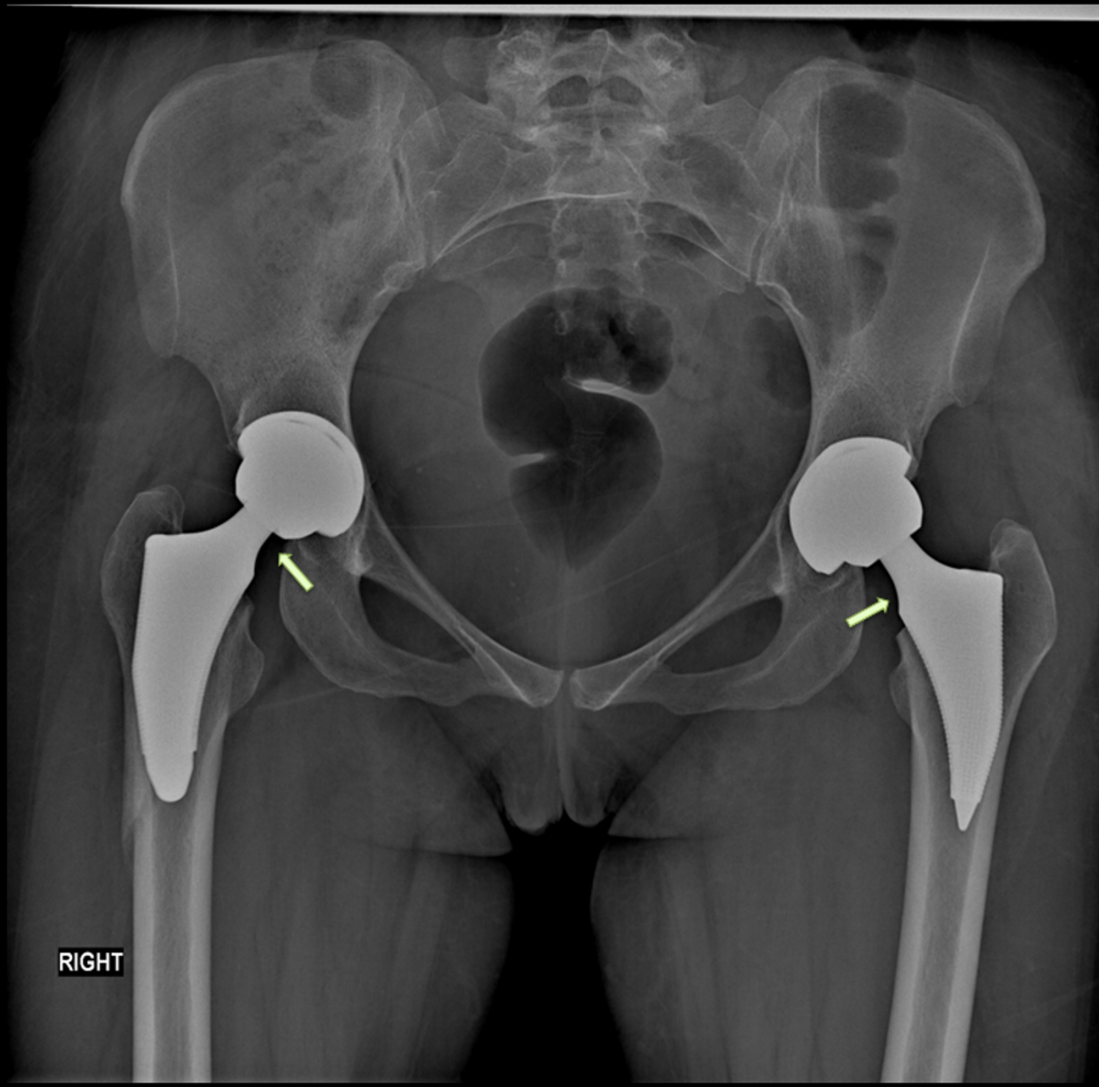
Postoperative radiograph of the pelvis showing bilateral total hip ceramic on ceramic replacement
